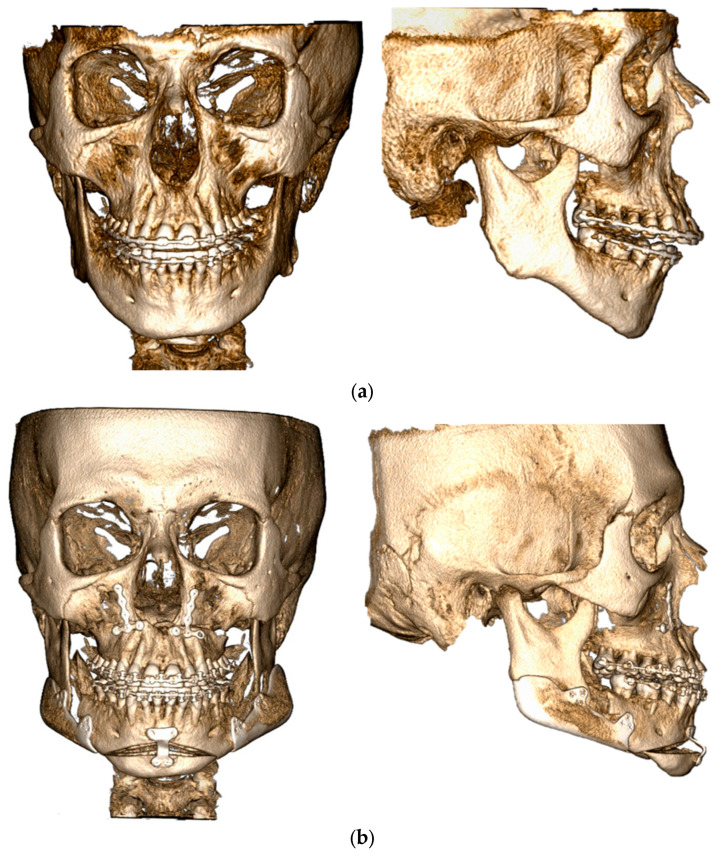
(a) Preoperative CT scan in frontal and lateral view; (b) one-month postoperative CT scan in frontal and lateral view.
